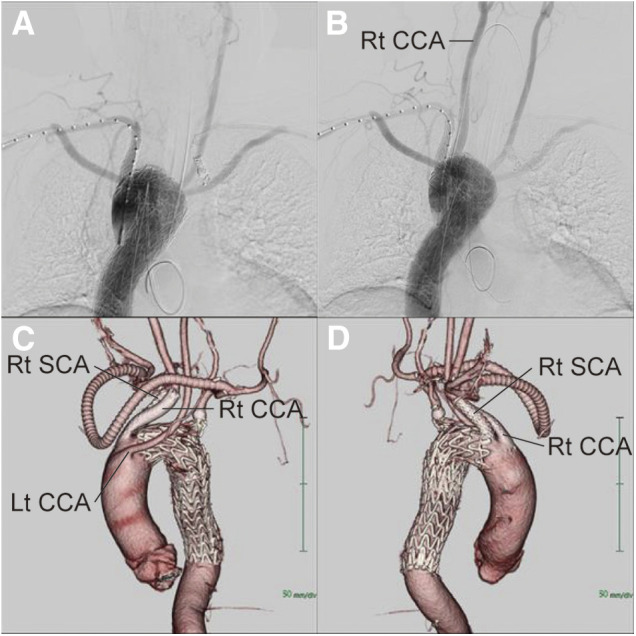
Fig. 2 Intraoperative aortography and postoperative CT. Intraoperative aortography before (A) and after (B) an additional stent placement at the right common carotid artery ostium. (C and D) Three-dimensional CT images prior to discharge. CT: computed tomography; Lt CCA: left common carotid artery; Rt CCA: right common carotid artery; Rt SCA: right subclavian artery
